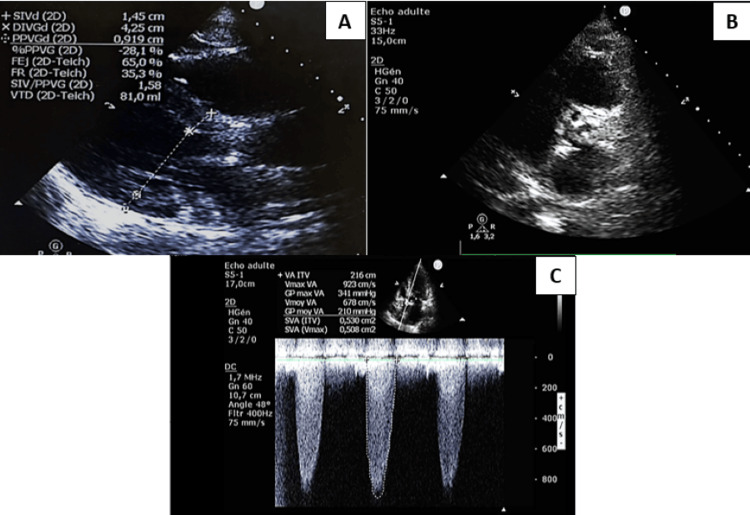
Transthoracic echocardiography showing concentric left ventricular hypertrophy(A) and a heavily calcified aortic valve (B) with overwhelming aortic stenosis parameters (C)
